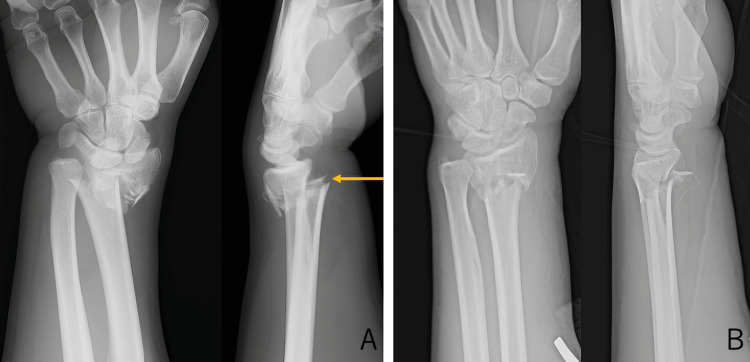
Preoperative radiographs of the left wrist A: Initial plain radiograph at presentation demonstrating a Colles-type distal radius fracture. A prominent radial volar third fragment is visible (arrow). B: Post-reduction radiograph obtained on the day of injury showing improved alignment after closed reduction
